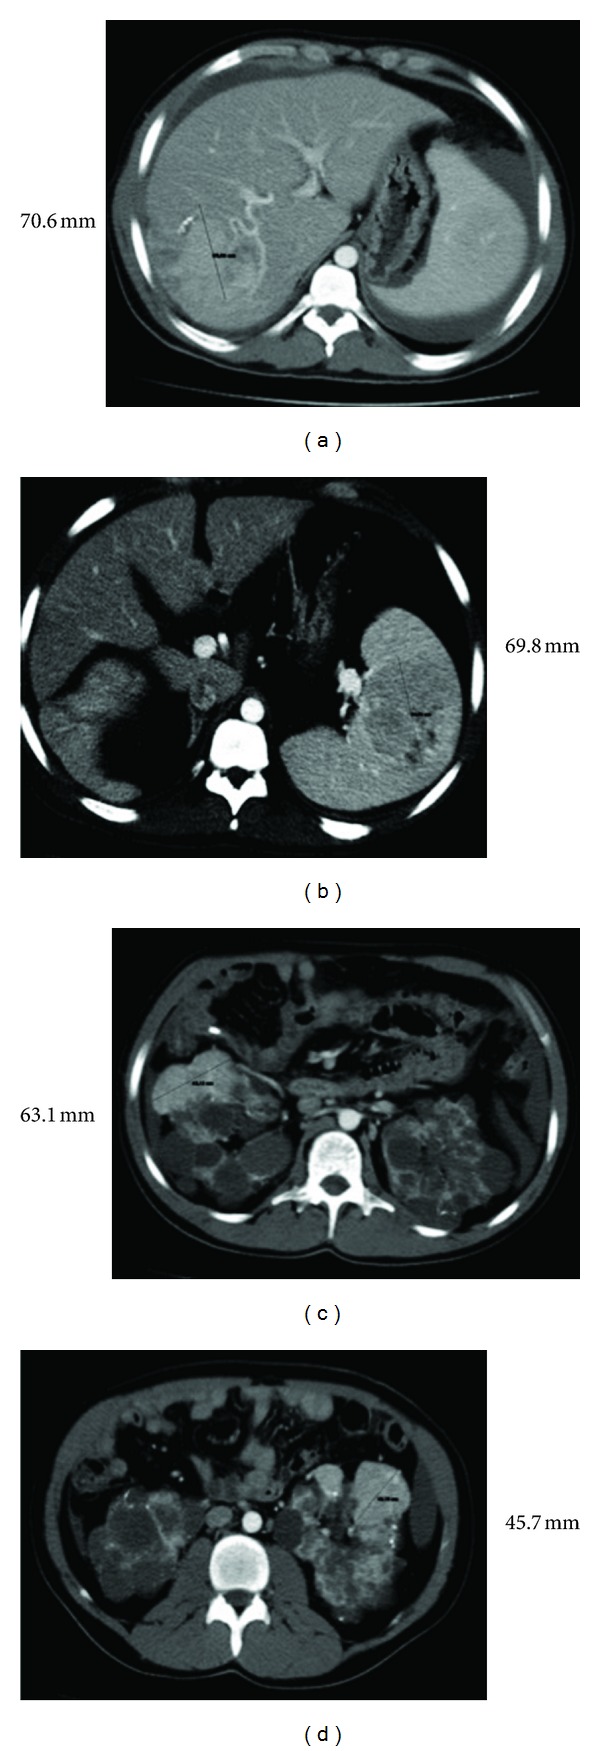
Abdominal CT (prior to renal transplantation) showing different lines of tumours in liver, spleen, and kidneys.
